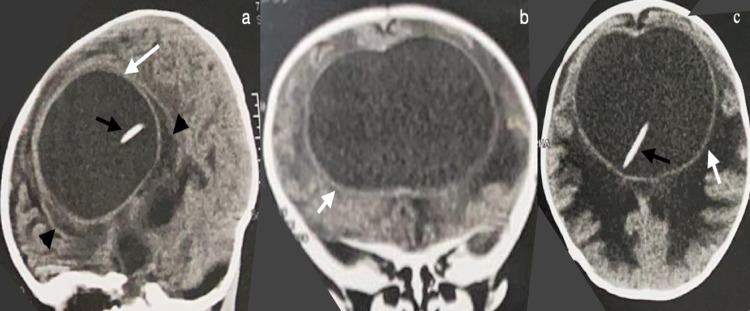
Computed tomography (CT) scan without contrast enhancement CT scan without contrast enhancement in the sagittal (a), coronal (b), and axial (c) planes. Shows pseudocyst (white arrows) inside the ventricular cavity (black arrowheads) and the catheter (black arrows).
